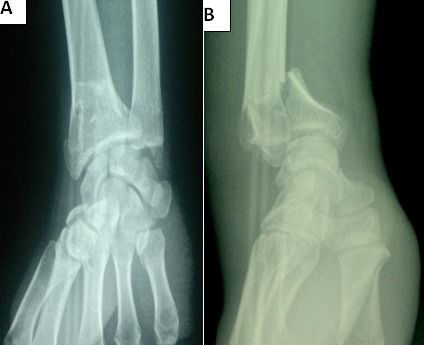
(A) preoperative anteroposterior radiograph of the left wrist joint, showing an intra-articular fracture of distal radius; (B) preoperative lateral radiograph of the right wrist joint, showing a volar displacement: Goyrand-smith fracture with sagittal articular fracture line
